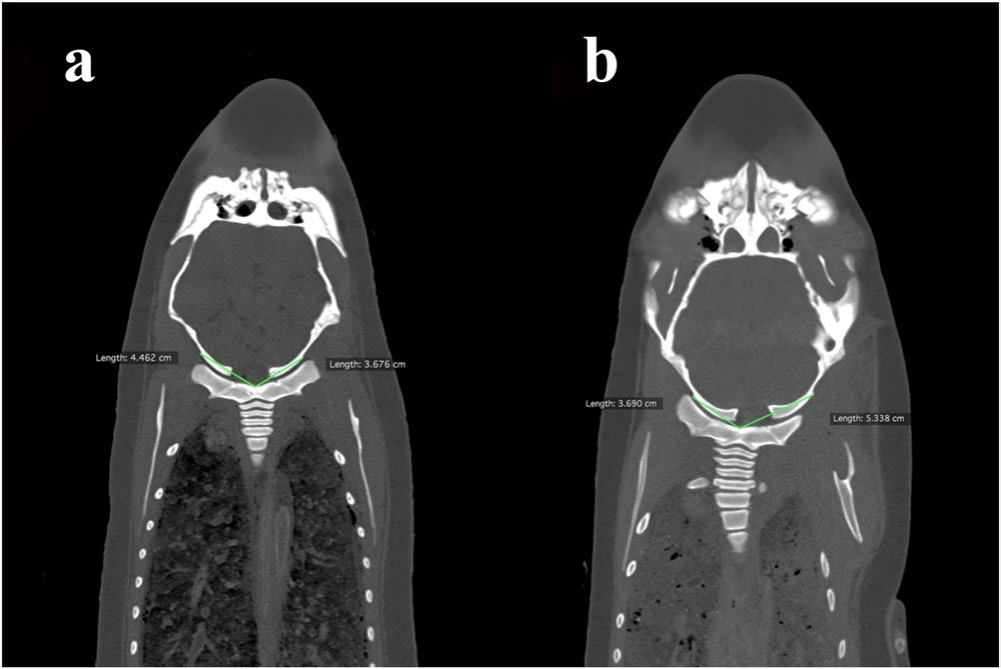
Reconstructed coronal postmortem computed tomography images of finless porpoise diagnosed with atlanto-occipital dissociation (AOD) in medial-lateral dimension, (a) right laterally displaced AOD and (b) left laterally displaced AOD.
